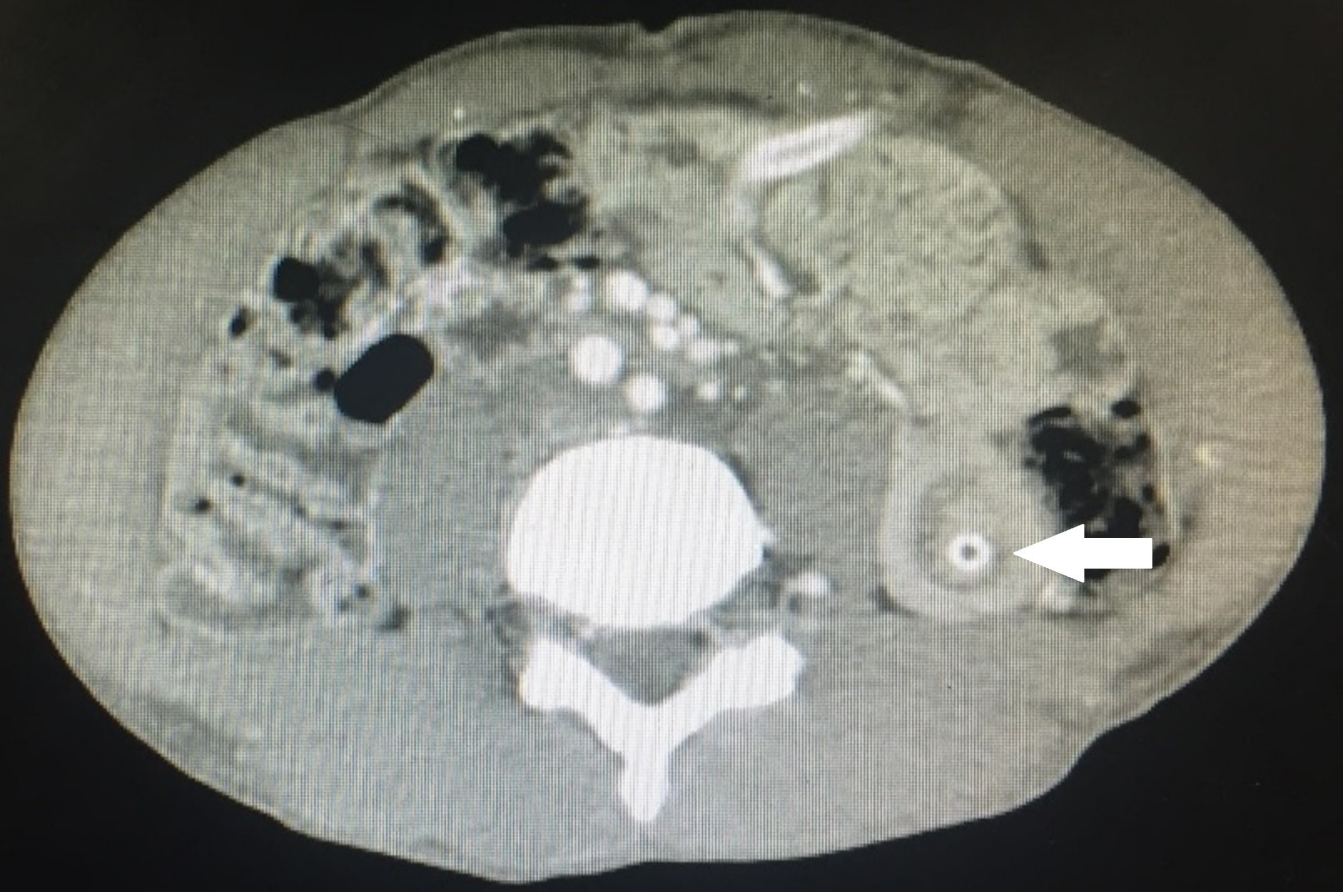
CECT showing the target sign of jejunojejunal intussusception (white arrow), which was conservatively managed CECT: contrast-enhanced computed tomography
